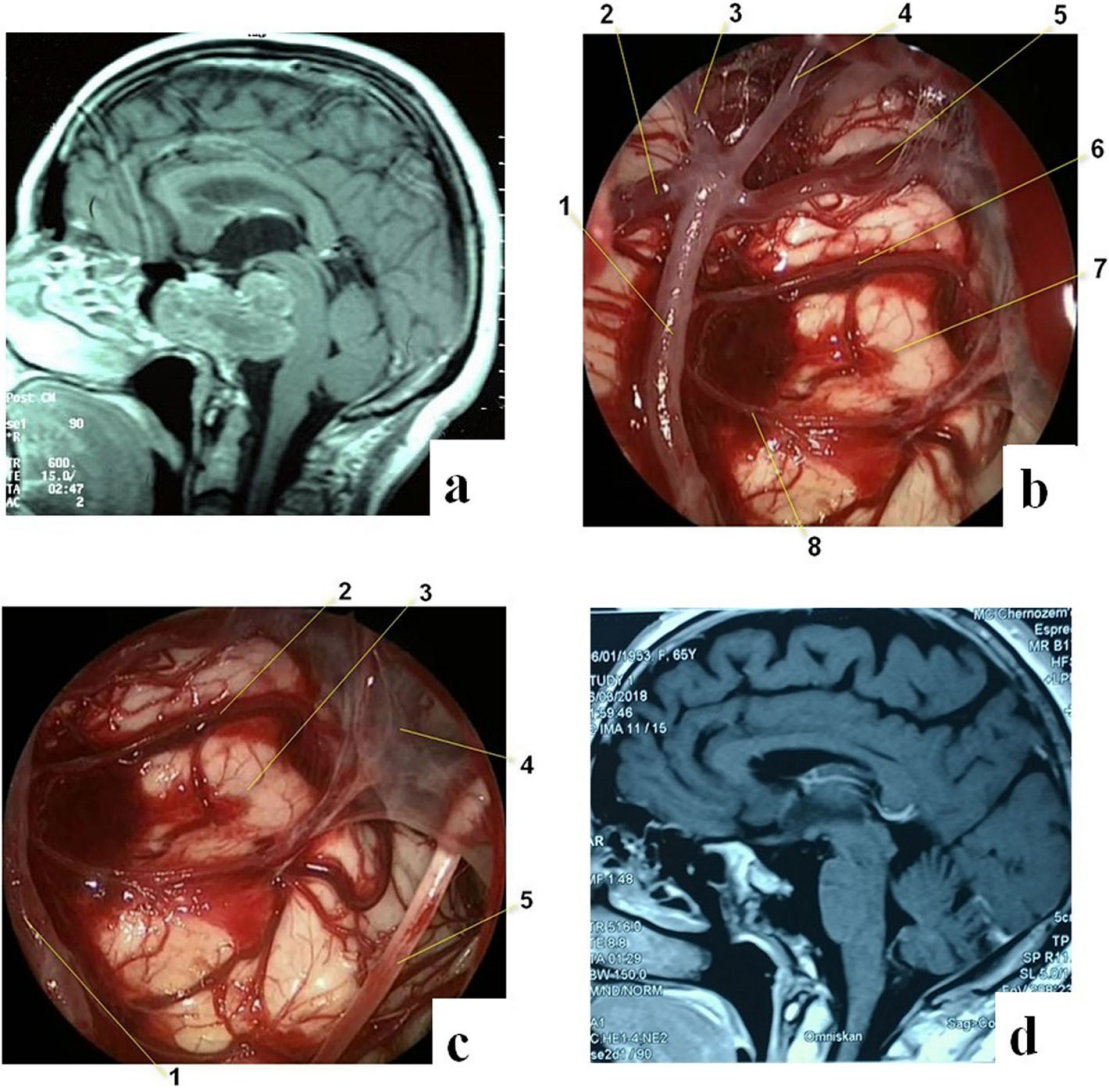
Clinical case 1. 57-year-old female patient with a large skull base chordoma, leading to severe brainstem compression. a Preoperative MRI. b Intraoperative photograph: 1—basilar artery; 2— right SCA; 3—right PCA; 4—left PCA; 5—left SCA; 6—left AICA; 7—lower pons; and 8—left PICA. c Intraoperative photograph: 1—basilar artery; 2—left AICA; 3—upper pons; 4—arachnoid mater; and 5—left abducent nerve. d MRI 7.5 years after surgery (no signs of tumor recurrence)
